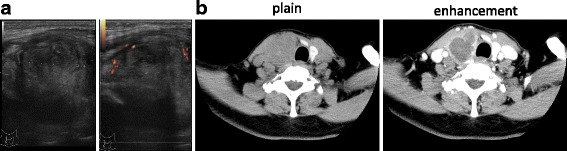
Ultrasonography on day X reveals a hypoechoic lesion with ill-defined margins and irregular form, appearing avascular and heterogeneous (a). Computed tomography of the neck on admission (day X + 5) also reveals a low-density lesion in the right thyroid gland, 37 × 37 × 42 mm in size with enhancement in the marginal area (b)
